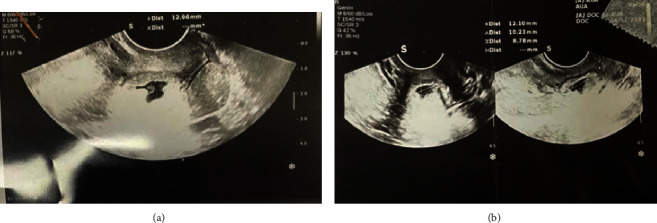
The 2^nd^ ultrasonography, a fetal echo (with FHR +) in the pregnancy bag.
